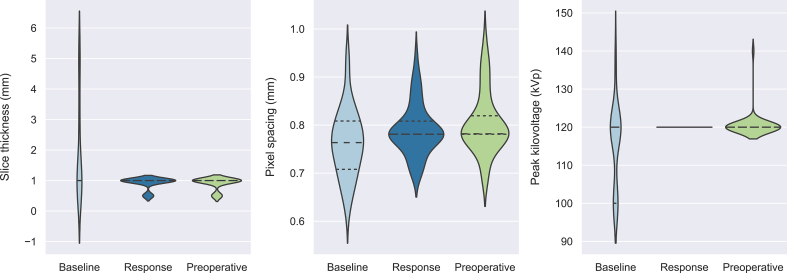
Violin plots visualising the distribution of acquisition parameters (slice thickness, pixel spacing and peak kilovoltage) for baseline (39 scans, 39 patients), interim response (54 scans, 30 patients), and preoperative (39 scans, 39 patients) portal venous CE-CT imaging. Baseline imaging was often performed in other hospitals, while response and preoperative imaging were both executed in a tertiary sarcoma referral centre. This has caused a heterogeneity within the acquisition protocols. The median value for slice thickness, pixel spacing and peak kilovoltage at baseline (1.0 mm, 0.76 mm, 120 kVp), response (1.0 mm, 0.78 mm, 120 kVp) and preoperative imaging (1.0 mm, 0.78 mm, 120 kVp) were computed. In baseline imaging (39 scans), four scanner manufacturers were observed including Siemens Healthineers, GE Healthcare, Canon Medical Systems (previously known as Toshiba Medical Systems) and Philips Healthcare. Response (54 scans) and final preoperative imaging (39 scans) were performed using only Canon Medical imaging systems.
